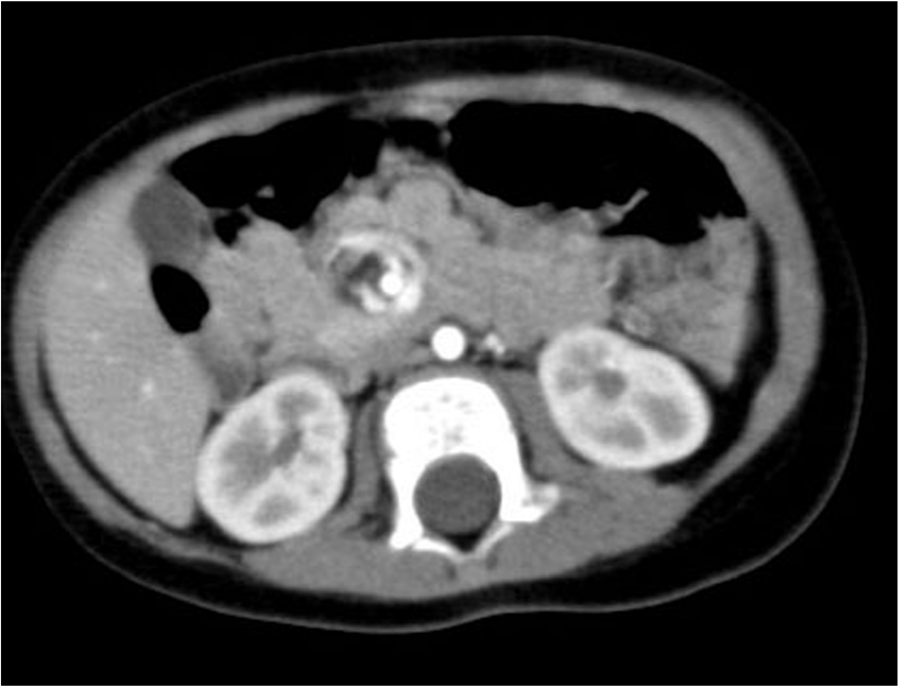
Contrast-enhanced CT: a whirlpool sign at the right upper abdomen. Neither intrahepatic portal venous gas nor signs of pneumatosis intestinalis were observed
